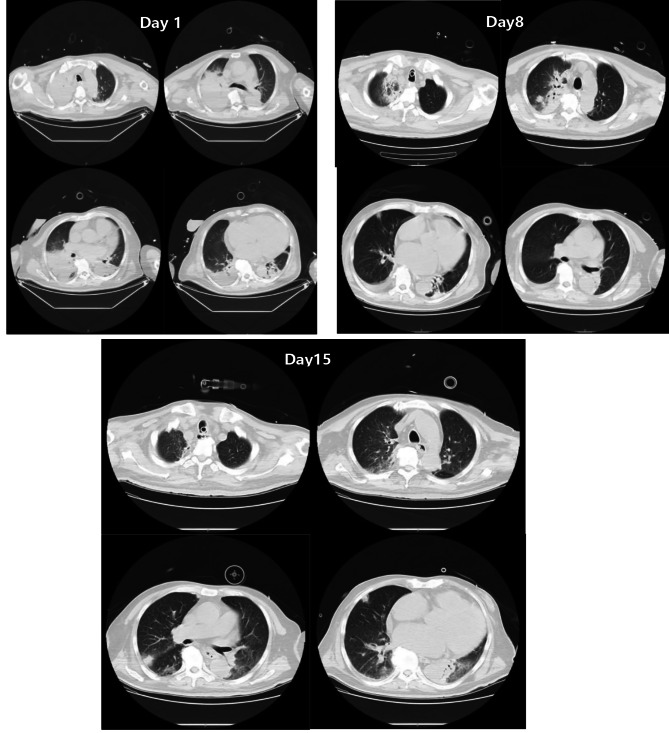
Comparison of the lung CT images of the patient during the ICU stay
